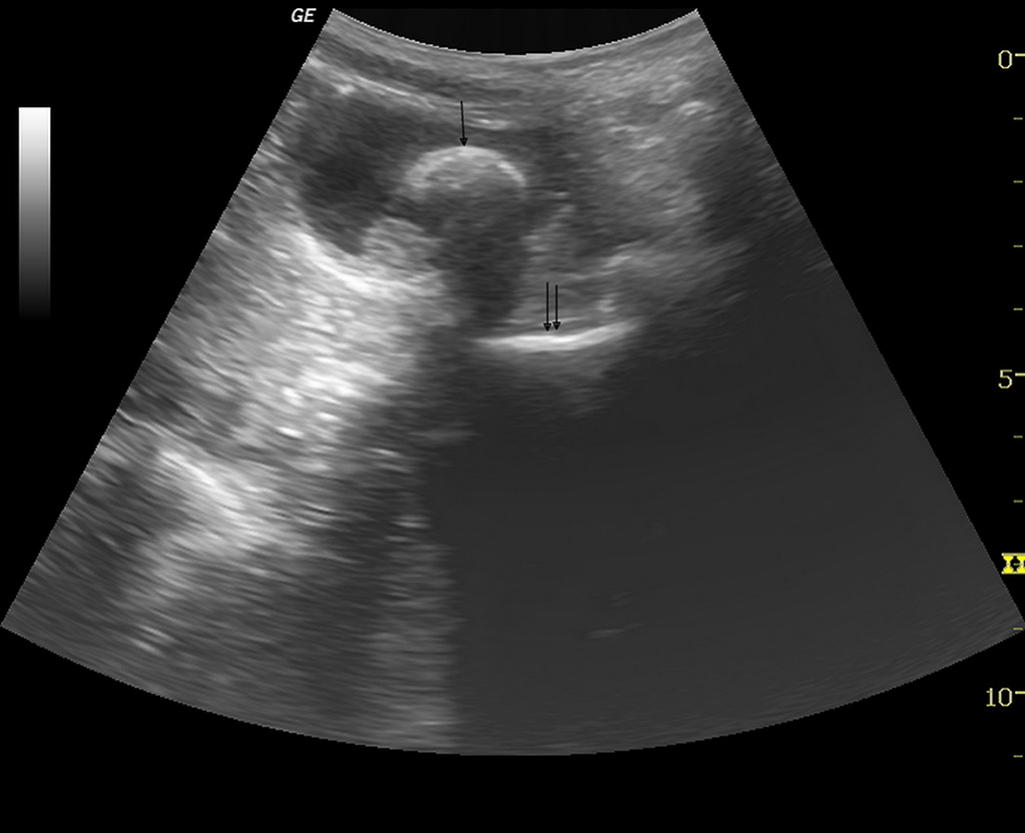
Sagittal sonogram of the pelvis showing a large calculus (arrow) in the lumen of the urinary bladder. Another echogenic shadow (double arrow) is seen posterior to the urinary bladder, suggestive of a vaginal foreign body.
